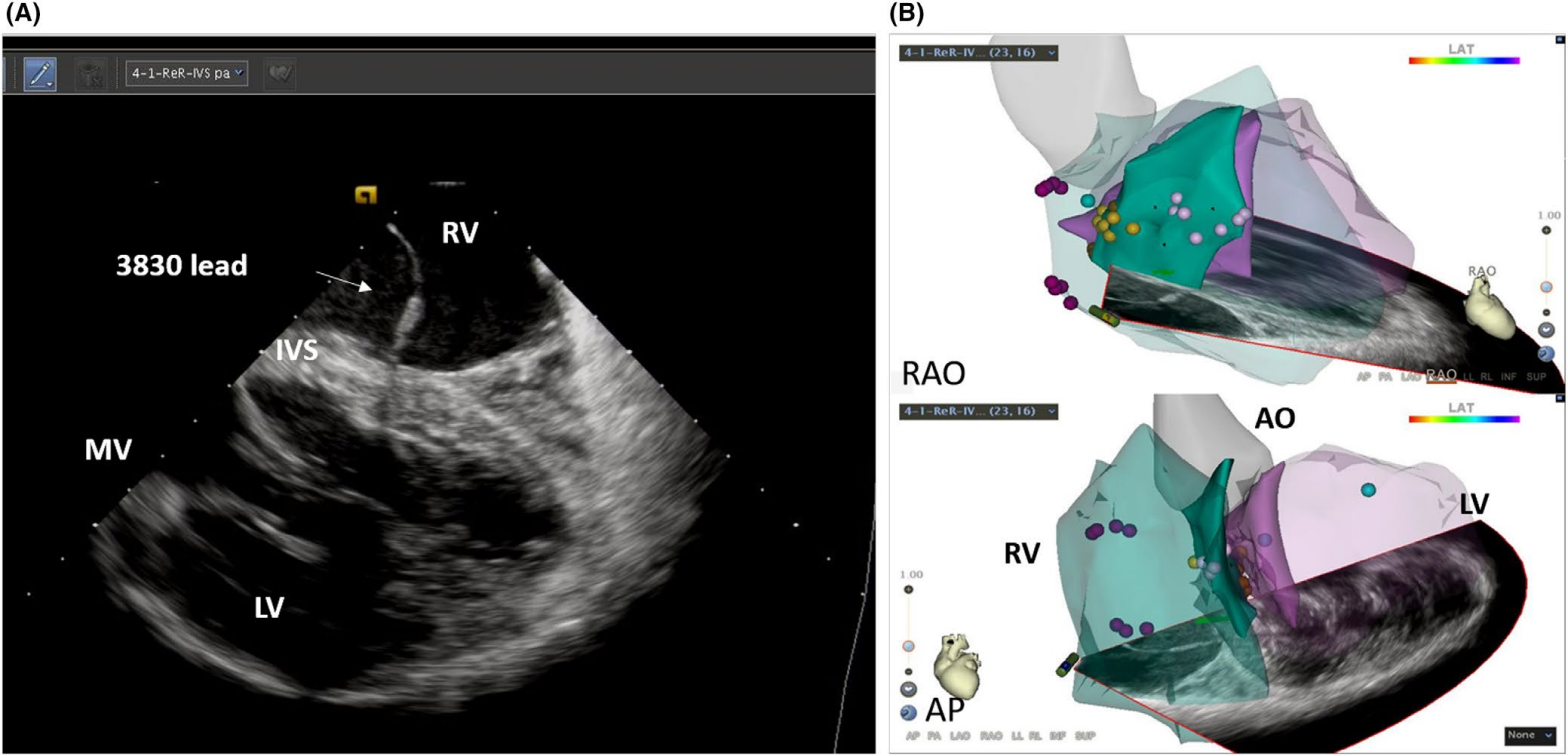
The initial site of the lead and the relationship of the interventricular septum in ICE. A, Intracardiac ultrasonic section showed the left and right ventricles and IVS, the 315 sheath and the initial site of the lead (small white arrow). B, The RAO and AP view showed the IVS model established by the ultrasonic catheter, the position of the ultrasonic catheter, and ultrasonic section. The ultrasonic catheter is located in the right ventricle and the ultrasonic section points to the septum. Abbreviations: AO, aorta; AP, anteroposterior; IVS, interventricular septum; LV, left ventricle; MV, mitral valve; RAO, right anterior oblique; RV, right ventricle
